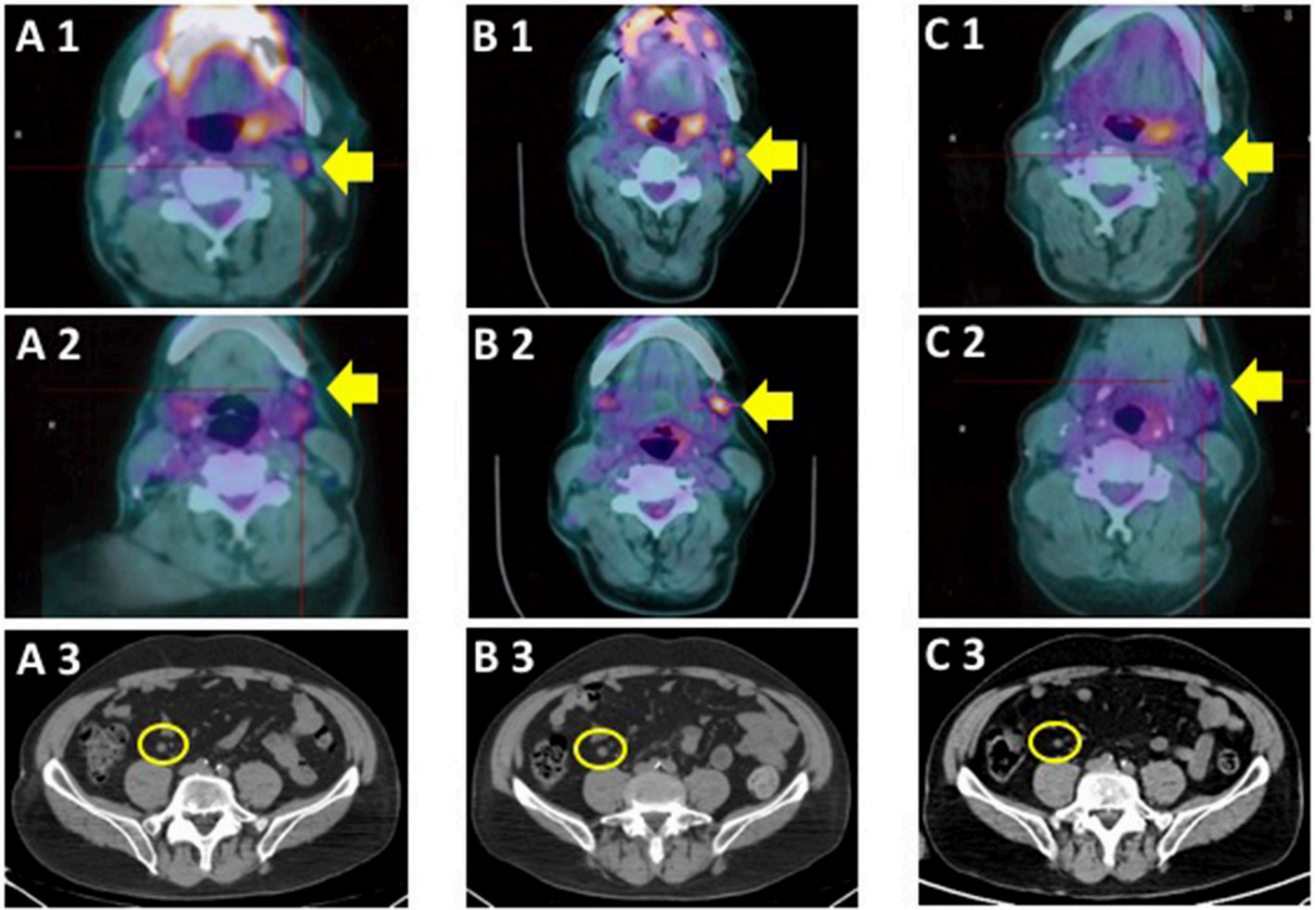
Comparison of ^18^FDG-PET/CT scan at baseline (A), first (B) and second (C) assessment during the treatment. 1: left retro-mandibular lymph node, indicated by yellow arrows; 2: left latero-cervical lymph node, indicated by yellow arrows; 3: nodule in the mesenteric adipose tissue in right hypogastrium, circled.
